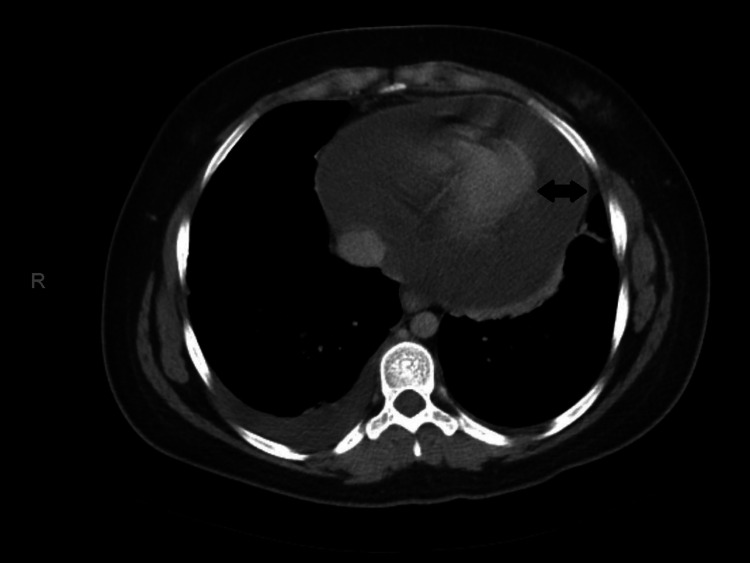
Axial view of the computed tomography of abdomen showing pericardial effusion
